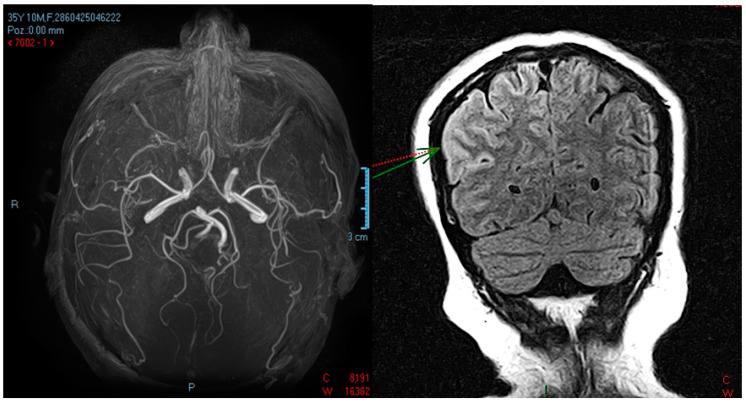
Three-dimensional Time-of-Flight (TOF) MR angiography: main endocranial arteries with fast flow and normal morphology. Asymmetry on the coronal FLAIR sequence (arrow).
